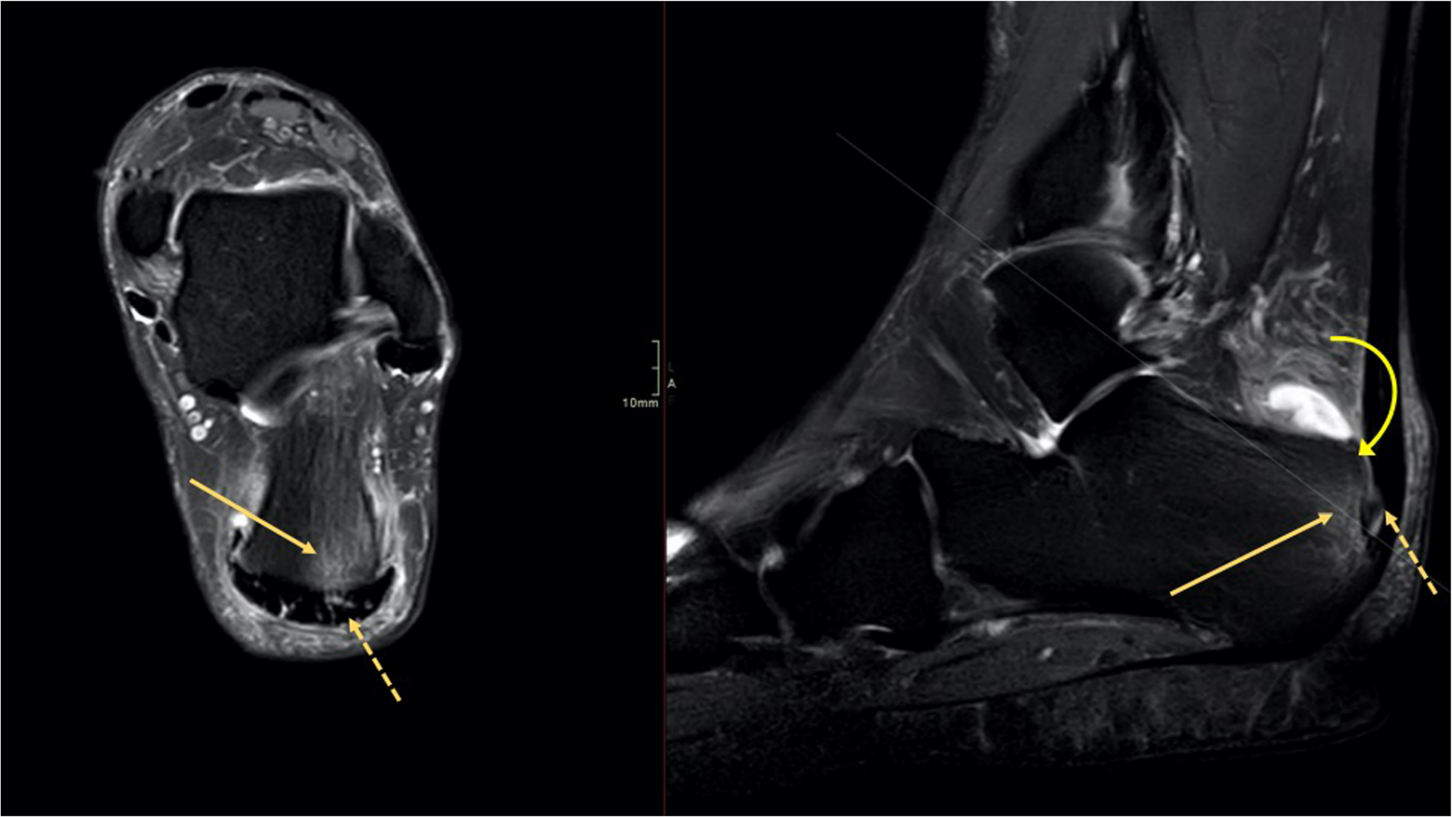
A 49-year-old male with chronic pain at the Achilles tendon enthesis and suspicion of a Haglund deformity. MRI showed enthesopathy of the Achilles tendon (dashed arrow) and BME in the tuber calcanei (arrow) without evidence of a Haglund deformity (curved arrow)
